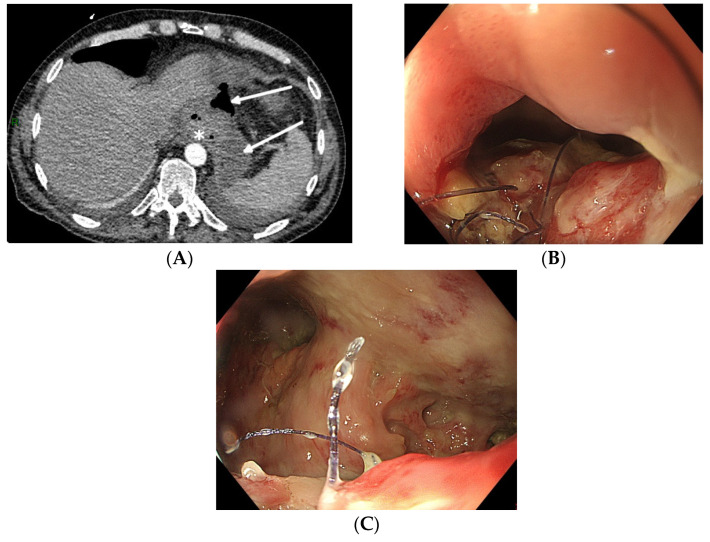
Total gastrectomy with an oesophagojejunal anastomosis complicated by an anastomotic leak. (A) Pre-procedure evaluation by CT scan (white arrows: hydroaeric collection; white star: anastomotic leak). (B) Pre-procedure endoscopic evaluation with inspection of the anastomotic leak. (C) Pre-procedure endoscopic evaluation of the extramural cavity.
